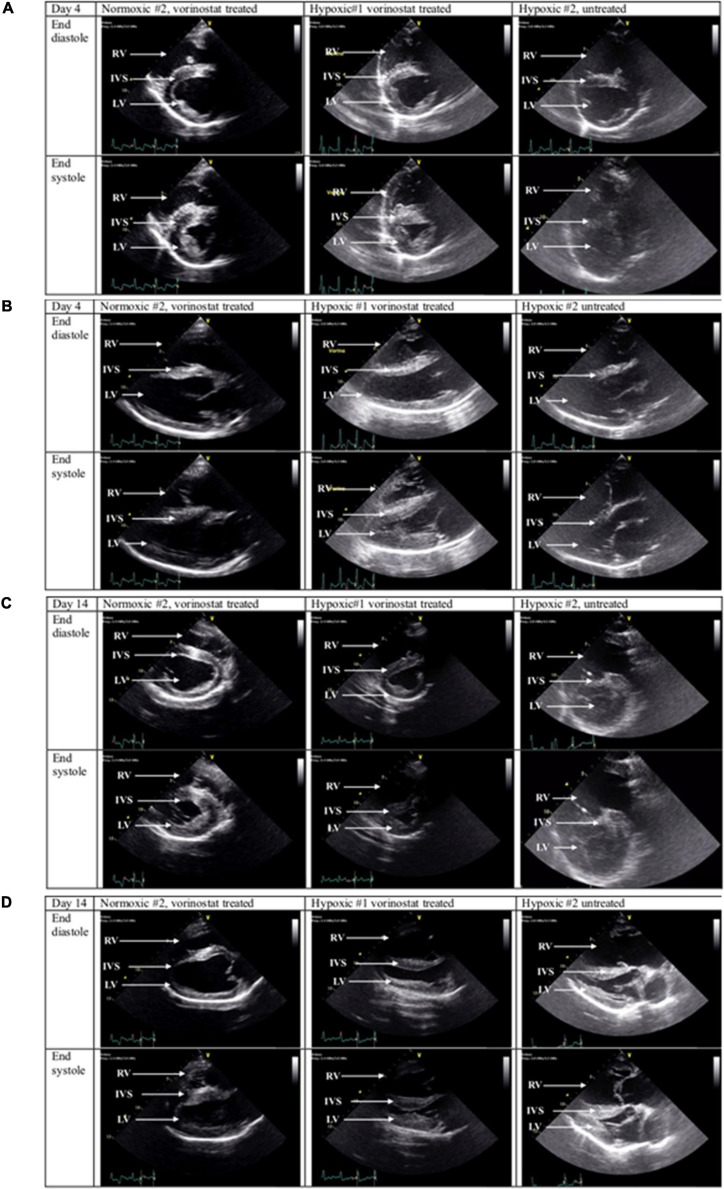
Echocardiographic imaging of vorinostat effects in calves with hypoxia-induced pulmonary hypertension. Calf environmental exposures, vorinostat treatments, and echocardiography were performed as described in section “Materials and Methods.” Representative echo images captured at systole and diastole are shown for one normoxic vorinostat-treated calf, one hypoxic vorinostat-treated calf, and one hypoxic untreated calf. Note: Representative echo recordings of cinematic loops are shown in Supplementary Videos 1, 2. (A) Experimental day 4, parasternal short axis views. (B) Experimental day 4, parasternal long axis views. (C) Experimental day 14, parasternal short axis views. (D) Experimental day 14, parasternal long axis views.
